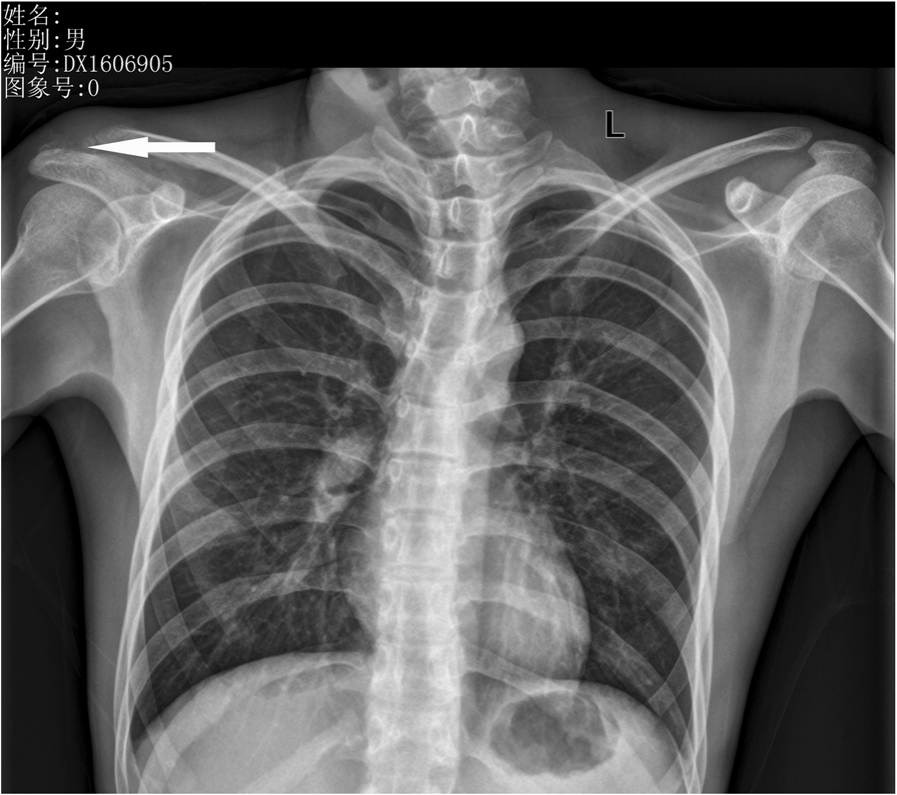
X-ray showing erosions at the acromion process of scapula
